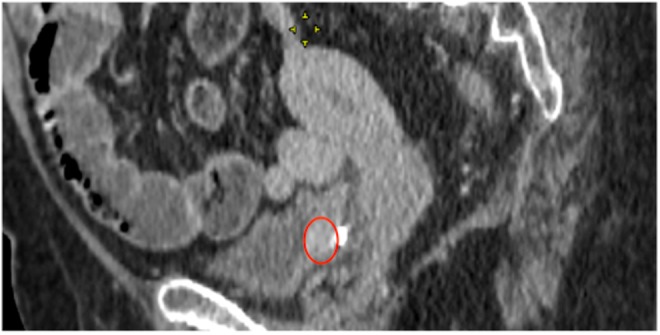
CT showing an enhancing mass (*red circle*) in the posterolateral right bladder wall.
